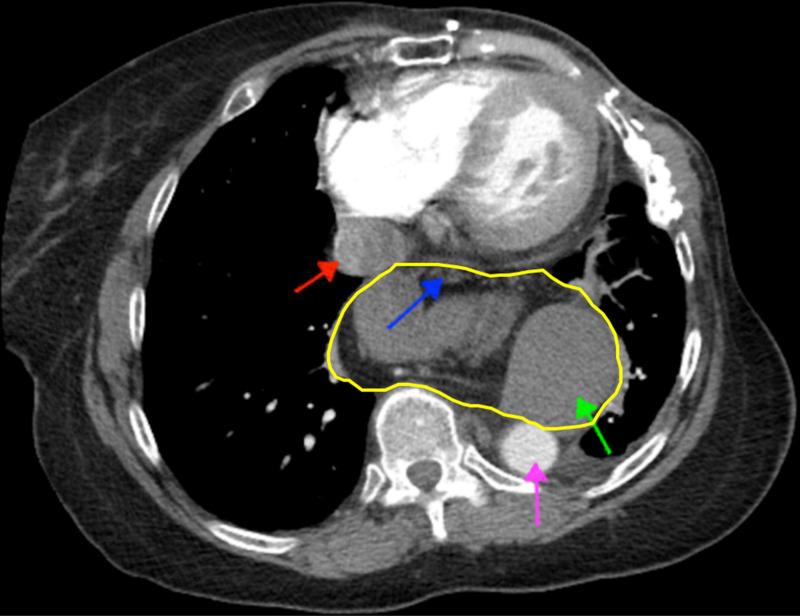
Axial CT angiogram of the chest featuring a distorted mediastinal anatomy and pleural cavity due to a massive hiatal hernia Retrosuperior displacement of the aorta (purple arrow) and inferior vena cava (red arrow) without compression of critical vasculature is appreciated, as the hiatal hernia with intra-abdominal contents (yellow outline) is seen protruding into the thorax. The intra-abdominal contents within the hiatal hernia include the pancreas and associated fat content (blue arrow) and stomach (green arrow).
